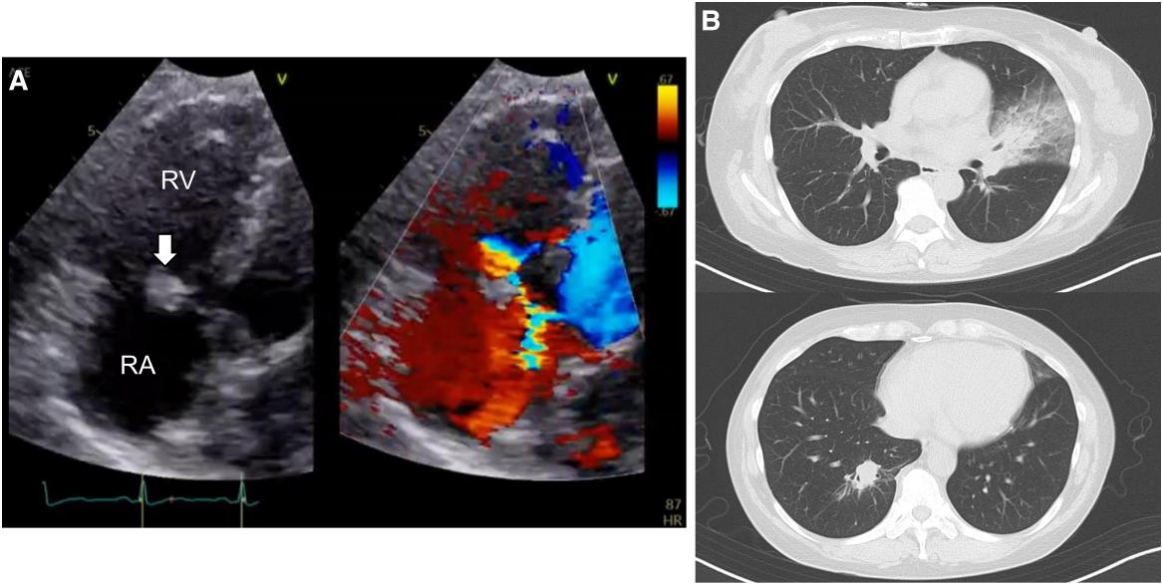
Chest computed tomography and transthoracic echocardiography indicated septic embolism in the lung due to tricuspid valvular infective endocarditis. (*A*) Transthoracic echocardiography showed vegetation (Thin arrow) was attached to the tricuspid valve concomitant with mild to moderate tricuspid valve regurgitation. (*B*) Chest computed tomography showed bilateral consolidation in the lingular segment of the left lung and the right lower lobe. RA, right atrium; RV, right ventricle.
